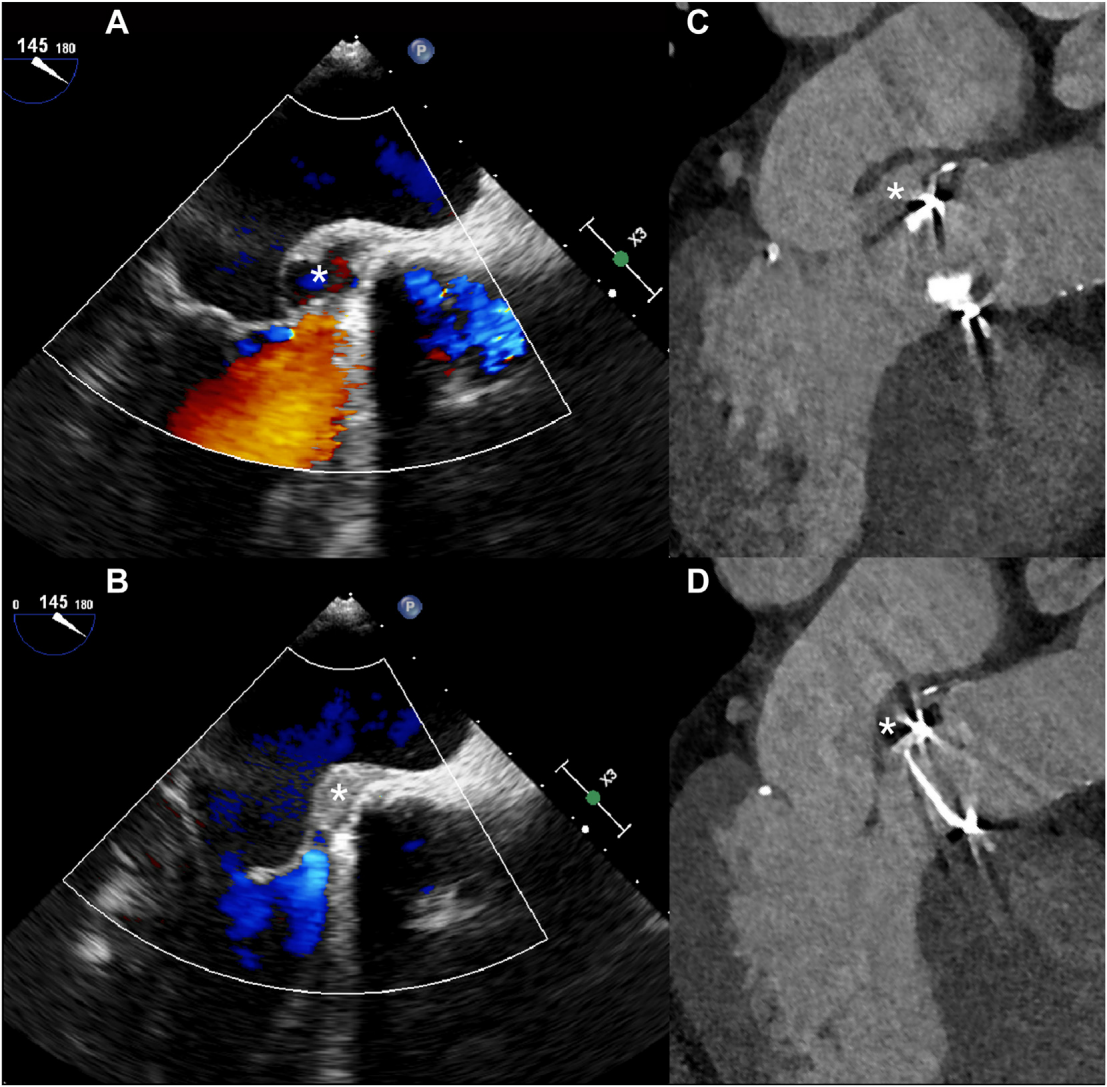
Aneurysm between the aortic root and left atrium. Aneurysm between the aortic root and left atrium filling with blood in the systolic phase and collapsing in the diastolic phase (asterisks) in a patient with endocarditis of a mechanical aortic valve shown on transesophageal echocardiography (A and B) and computed tomography (C and D).
